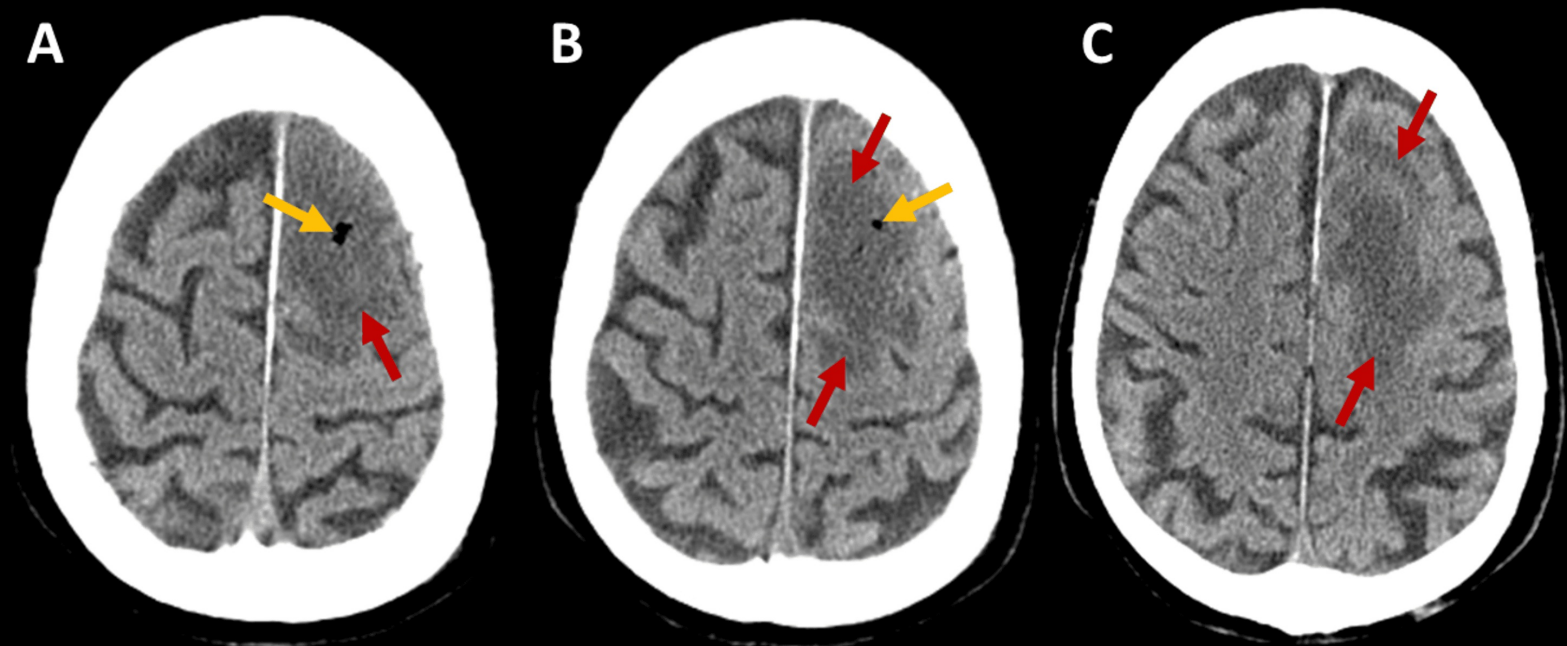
Brain CT scan in multiple axial planes (A-C) revealing an increase in the size of the hypodense lesion in the left frontal lobe (red arrows) with a reduction in the gaseous content (yellow arrows).
